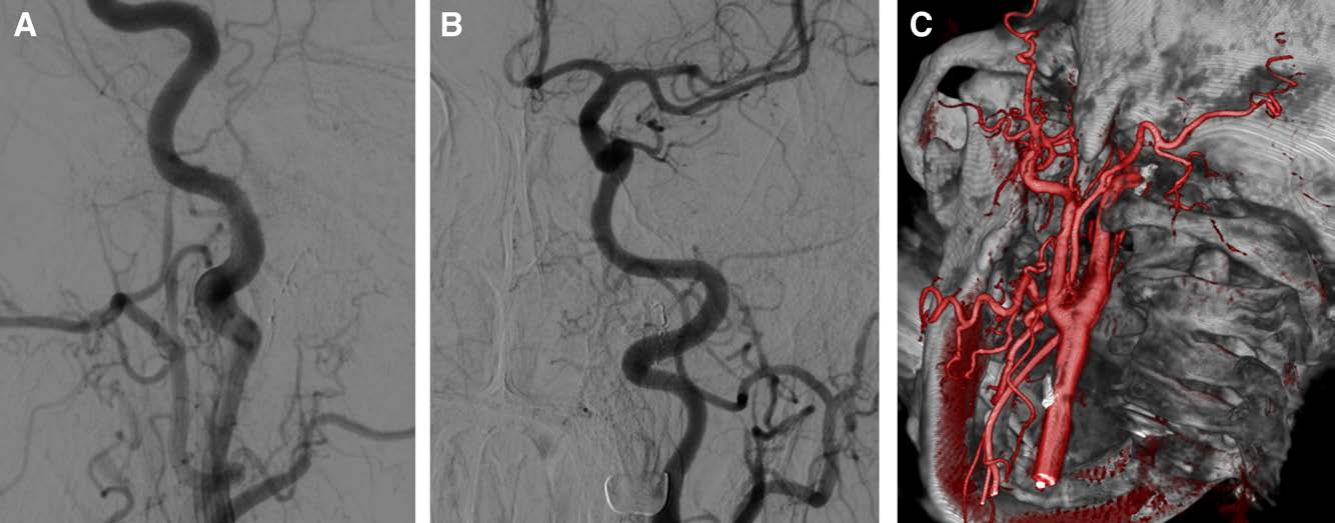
Review after surgery. (A, B) Postoperative, no abnormal venous (arrow). (C) Postoperative Xper-ray CT plain scan and preoperative 3-D reconstruction fusion showed that the glue was located in the jugular foramen (arrow).
